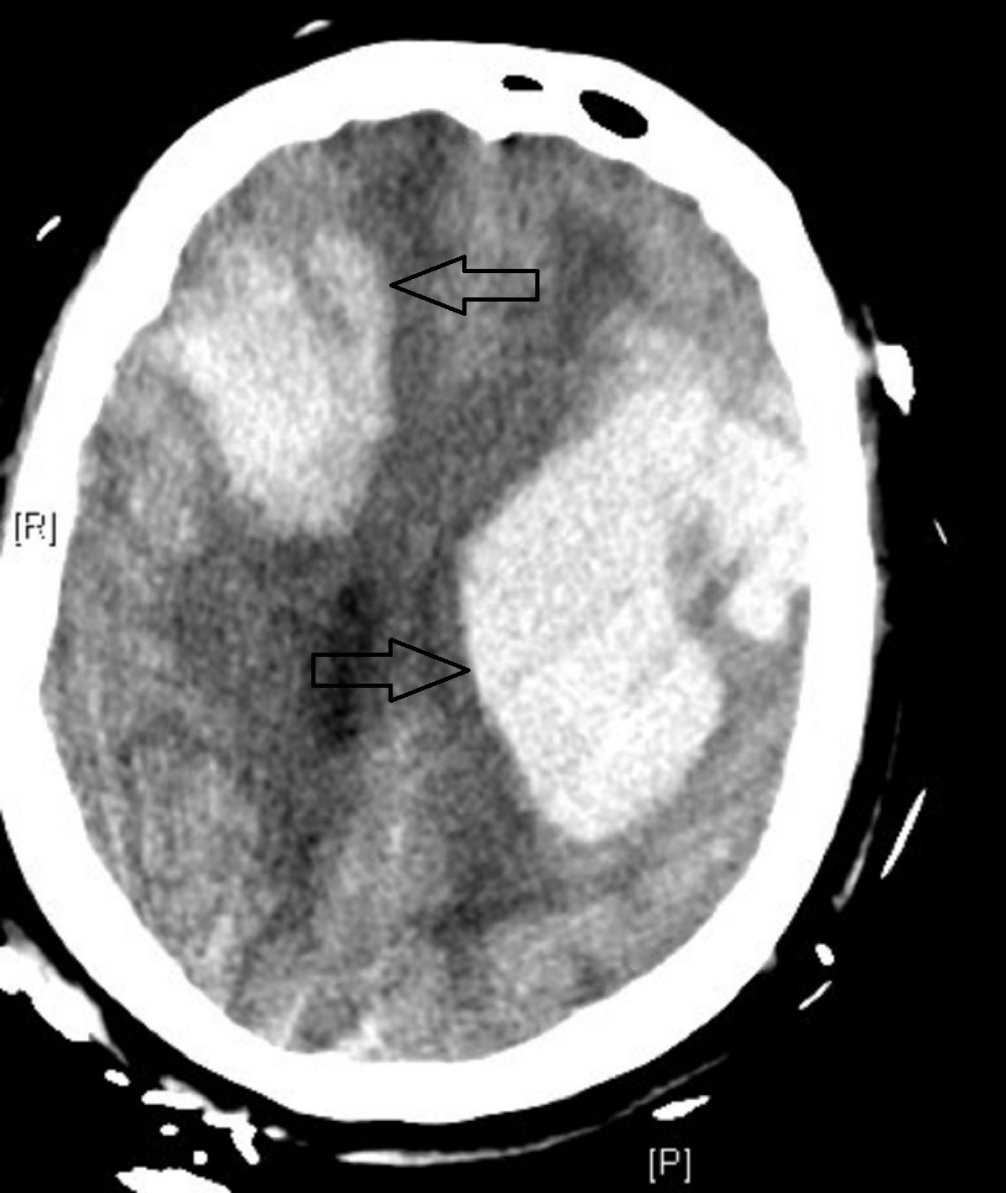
Computed Tomography (CT) Head on Day 9 CT shows the known right frontal intracerebral hemorrhage, but now with new left frontal intracerebral hemorrhage  (47.8 cc).
